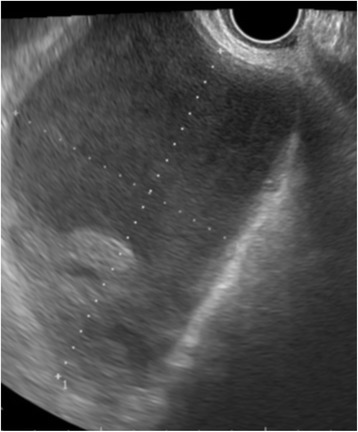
Transvaginal sonography revealed cystic lesions with septum at the bilateral ovary
